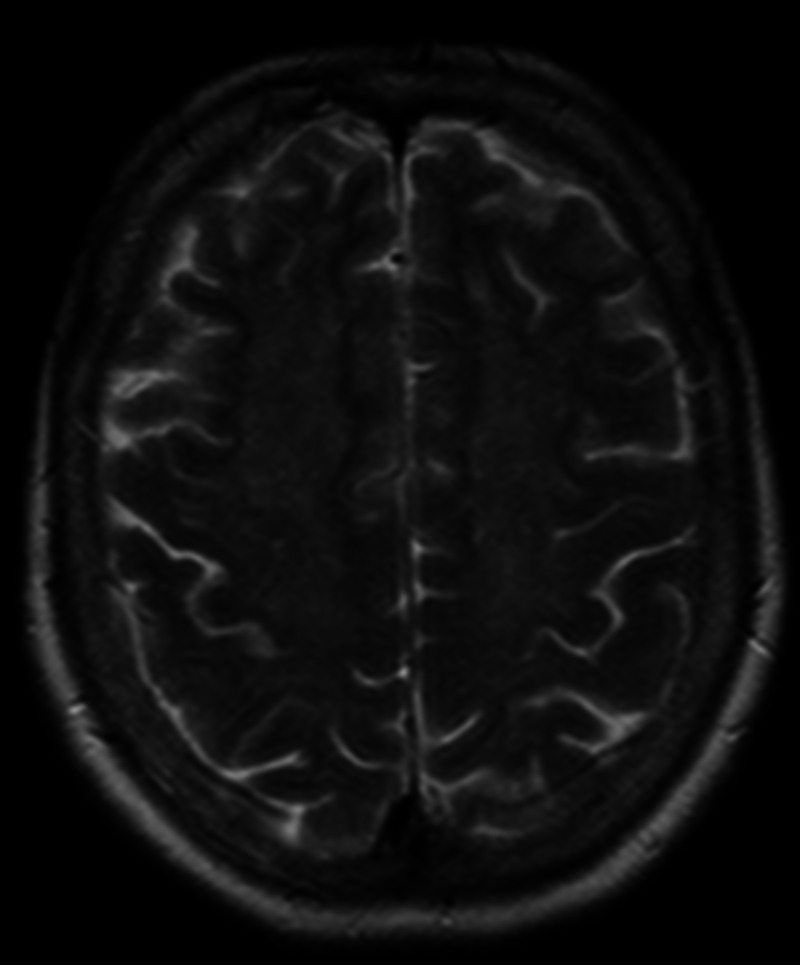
T2 weighted Axial MRI of the brain showing diffuse symmetric increased intensity signals throughout the white matter in frontal and parietal lobes
